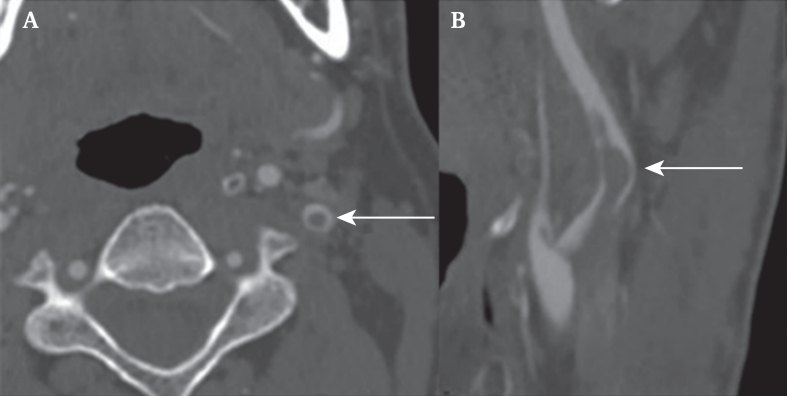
Computed tomography angiography demonstrating a free floating carotid thrombus in the left internal carotid artery with (A) the “doughnut sign” on the axial plane, and (B) the “finger sign” on the coronal plane, both indicated by the arrow.^24^
